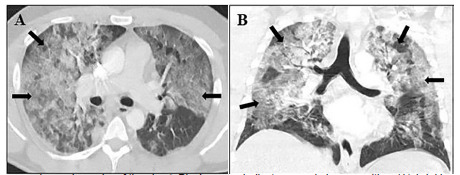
Computed tomography angiography of the chest. Black arrows indicate ground glass opacities. (A) Axial image showing bilateral central and peripheral ground-glass opacities. (B) Coronal image demonstrating bilateral ground-glass opacities in upper and lower lung fields.
